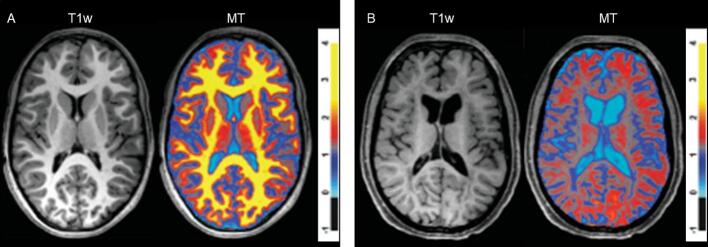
A) Normal magnetization transfer (MT) findings in an 11-year-old healthy control. B) Decreased MT ratios are visible in the cerebral white matter of a 13-year-old subject with the leukodystrophy Hypomyelination and Atrophy of Basal ganglia and Cerebellum, while the T1-weighted image still shows a relatively normal hyperintense T1-signal. Adapted with permission from Dreha-Kulaczewski et al., 2012.
